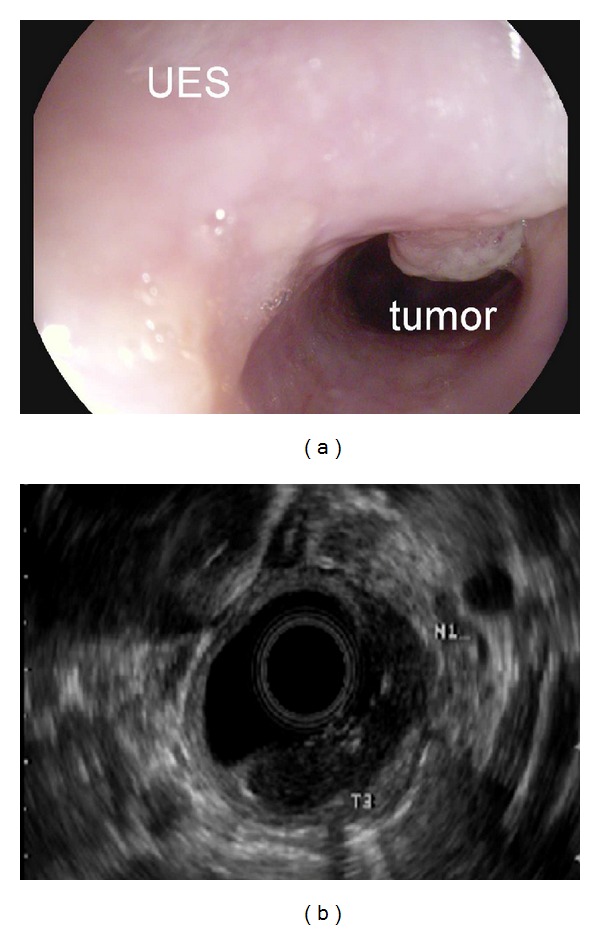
(a) Upper endoscopy highlights the close proximity of the tumor to the upper esophageal sphincter (UES). (b) Endoscopic ultrasound shows invasion of the tumor into the muscularis propria and suspicion for regional node involvement.
